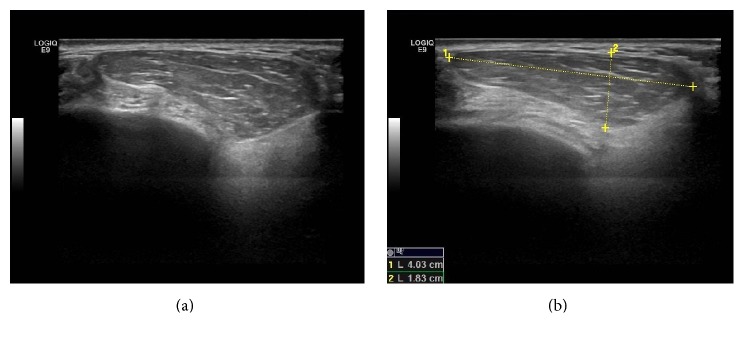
(a) Ultrasound image of the patient showing a well-defined, hypoechoic solid lesion in her superficial lobe of the parotid gland. The lesion had echogenic septas and acoustic empowerment over the posterior region. (b) The lesion was measured approximately 40 × 18 mm in size.
